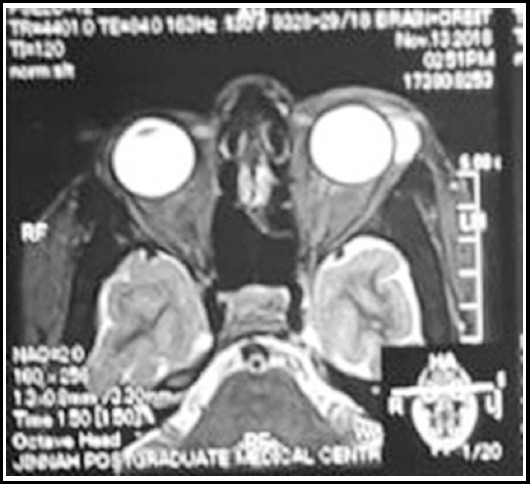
Magnetic resonance imaging (T2) shows hyperintense mass on left lateral aspect.
